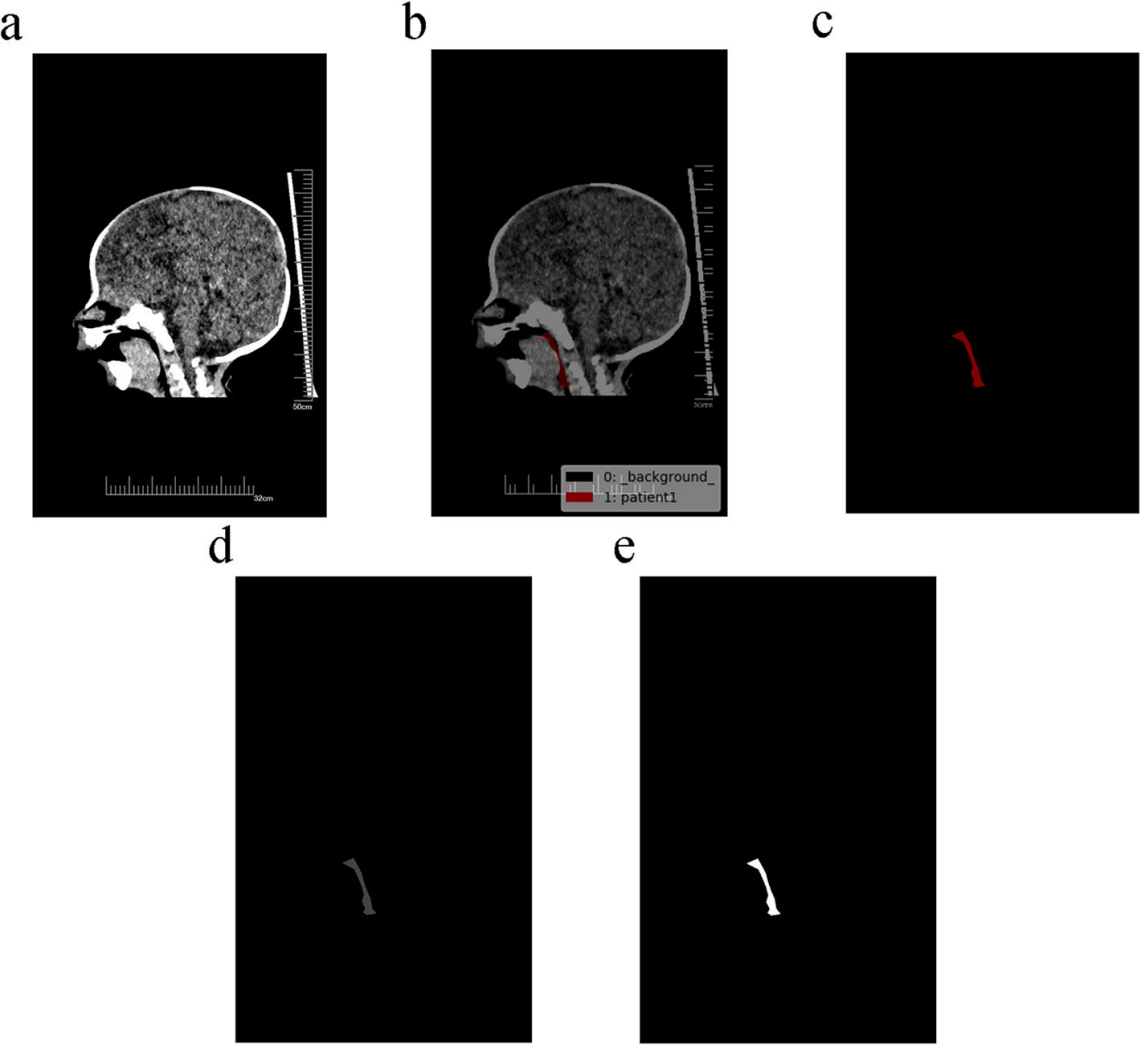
Images generated during area calculation. a Original CT image. b The image after labeling by labelme. c The *.png* image obtained by single-channel conversion. d The grayscale image obtained by color space conversion. e The binary image obtained after thresholding is performed
